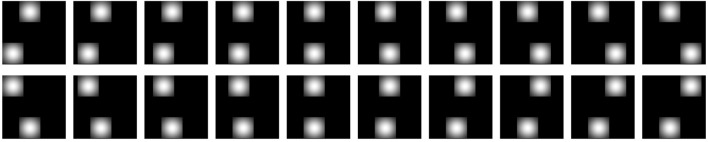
The layout prototypes used in conjunction with *CT* to encode the spatial structure of scenes (see section 7.1). There are two different sets of such prototypes. One set of 10 prototypes is used for encoding the scene when the top ROI is fixated; the other set of 10 prototypes is used when the bottom ROI is fixated. For each situation (scene + fixation), the scene structure was thus represented by a 10-dimensional vector of similarities between the layout of the scene's ROIs and the 10 layout prototypes.
